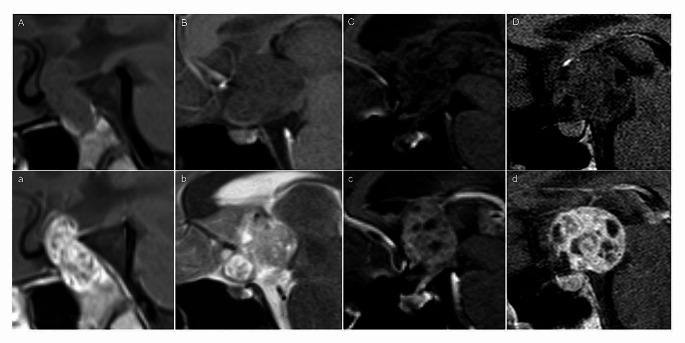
The upper row demonstrates the four cases with a preserved posterior pituitary bright spot on sagittal T1-weighted images without contrast. The cases are named as in Table 2. Especially case D shows an irregular bright spot. The lower row shows the tumor appearance in post-contrast T1-weighted images (a, c + d) and T2-weighted image (b; in that case, no post-contrast sagittal T1-weighted image was available)
